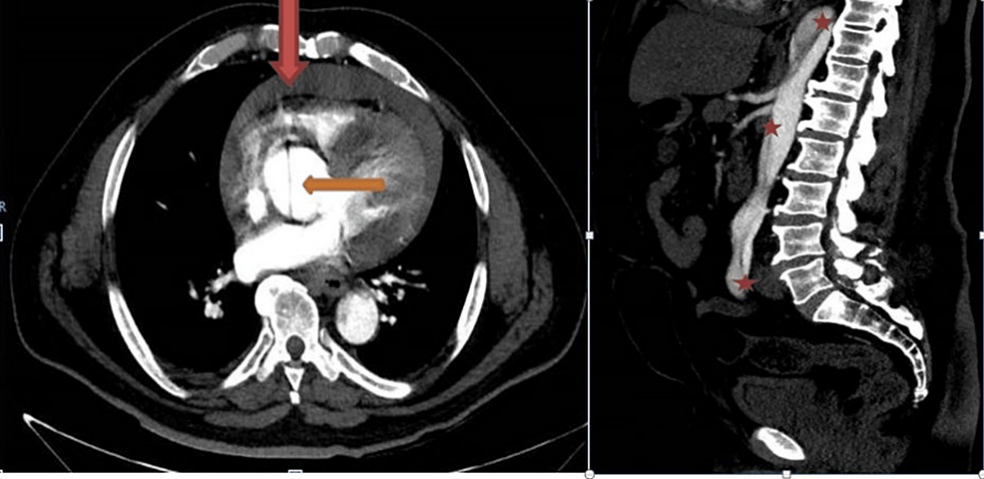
Left: Axial view of computed tomography; red arrow showing hemopericardium; Orange arrow indicating dissecting aorta. Right: Sagittal computed tomography section; three red stars mark the dissection line.
